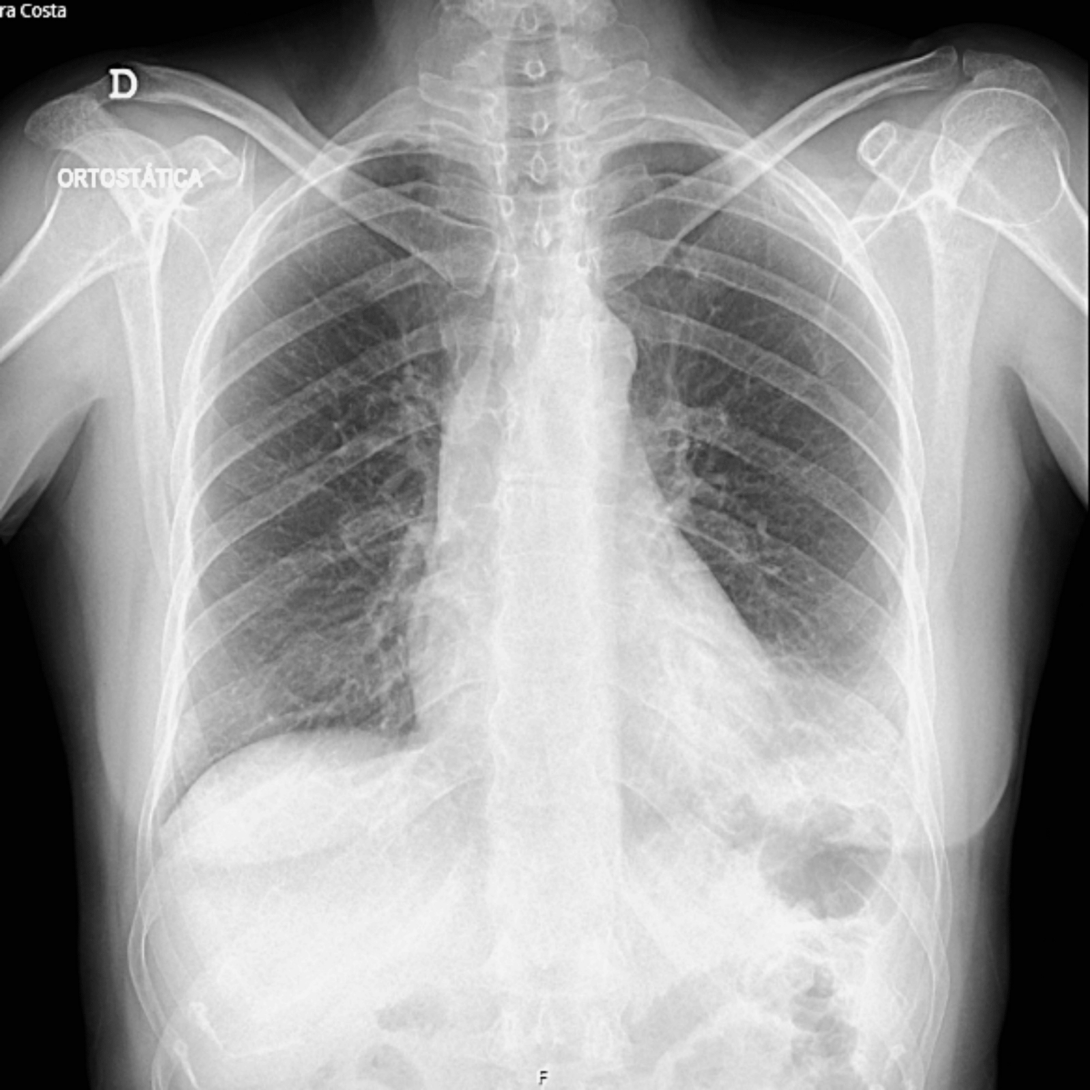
Chest X-ray performed one week earlier
